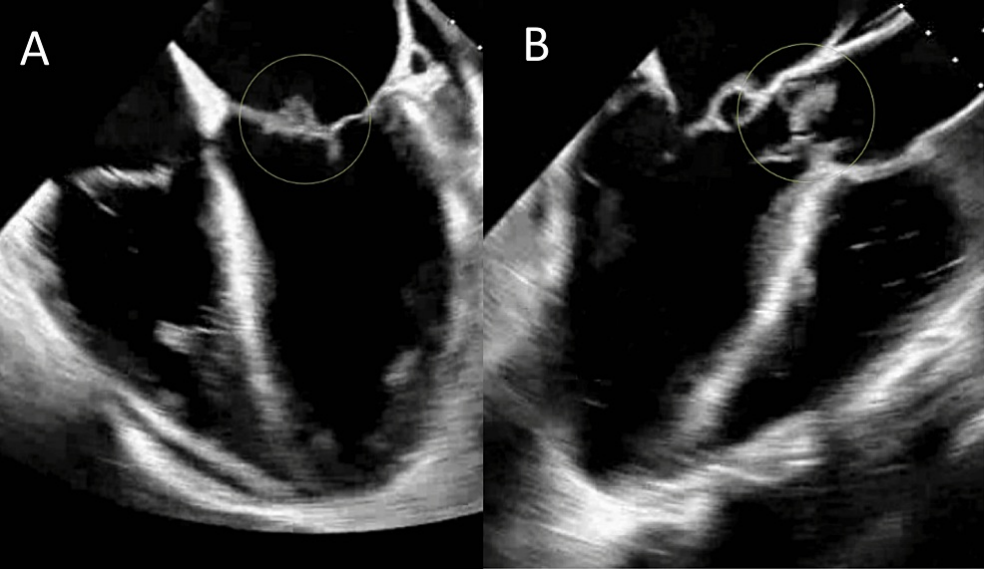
Vegetations of the mitral valve in picture (A) and aortic valve in picture (B) seen on the echocardiogram shown by circles around the vegetations.
